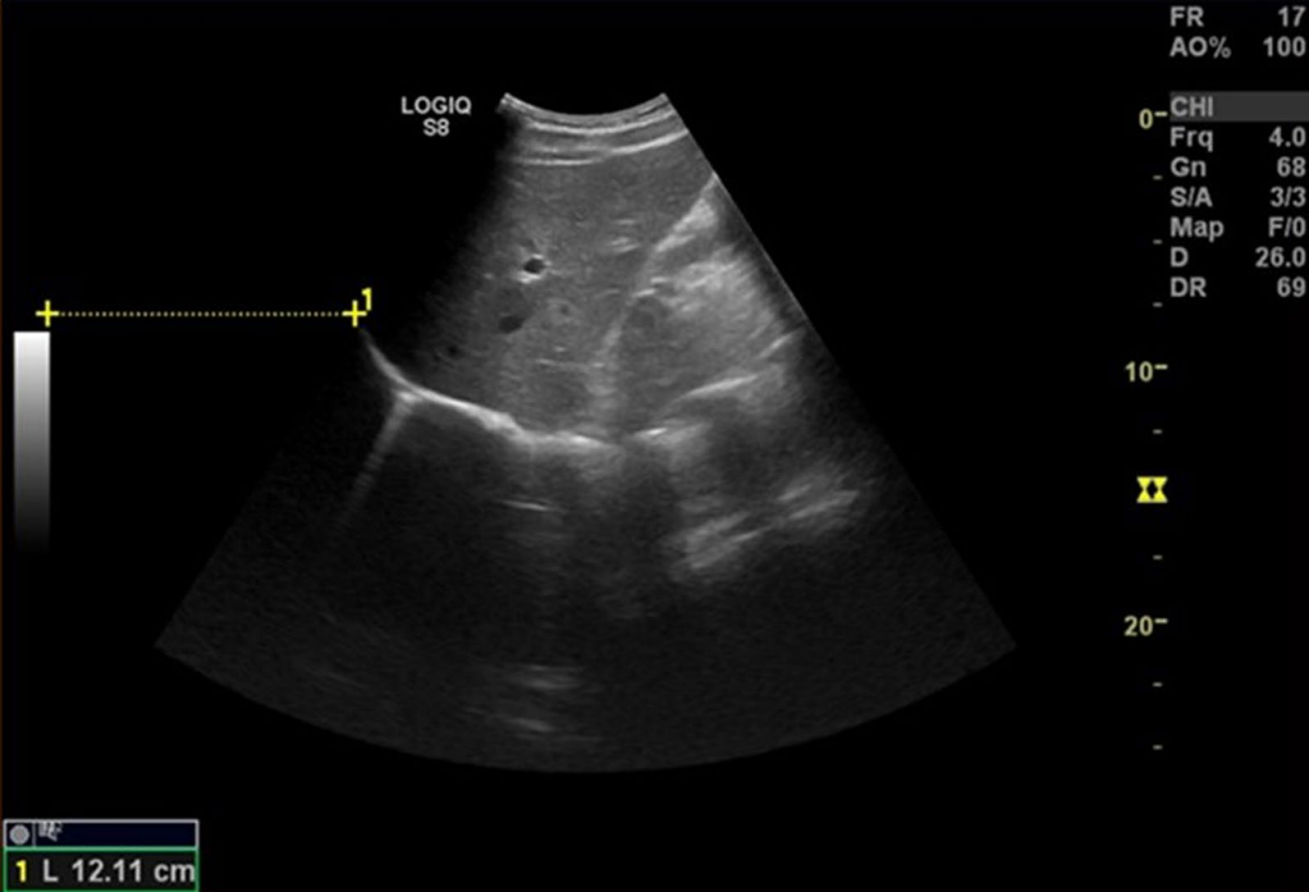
Lateral ultrasound view in the mid-axillary line assessing the cranio-caudal diaphragm movement with B-mode using ultrasound machine’s inbuilt distance measure function
